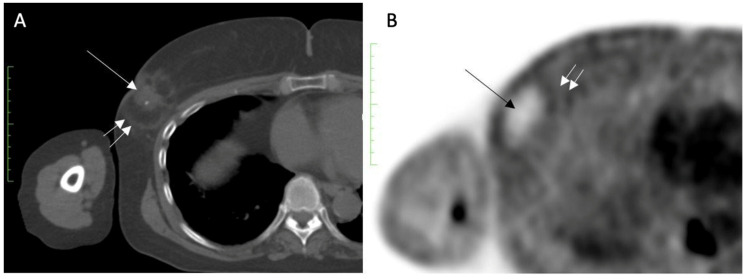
(A) shows an FDG-PET/CT performed 1 month after cryoablation of a 3 cm ER+, PR+, HER2/neu negative invasive ductal carcinoma, with a central tumor ghost (single arrow) and edge of spherical cryoball (double arrow). (B) shows a significant reduction in FDG-PET/CT at the cryoablation site (single arrow) compared to background levels of activity in the normal surrounding tissues (double arrow).
